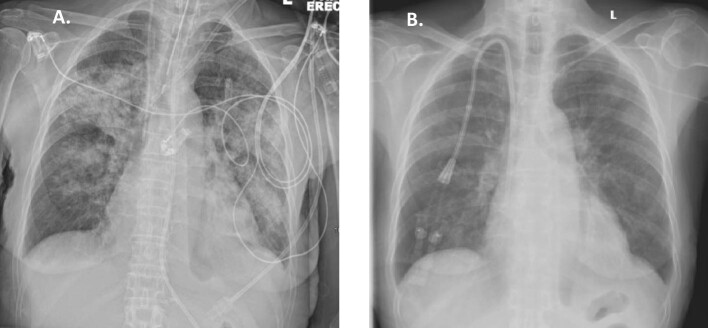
(A) Patient 3 at presentation: chest X-ray: bilateral consolidation; (B) Patient 3 after 10 days: chest X-ray: significant improvement.
